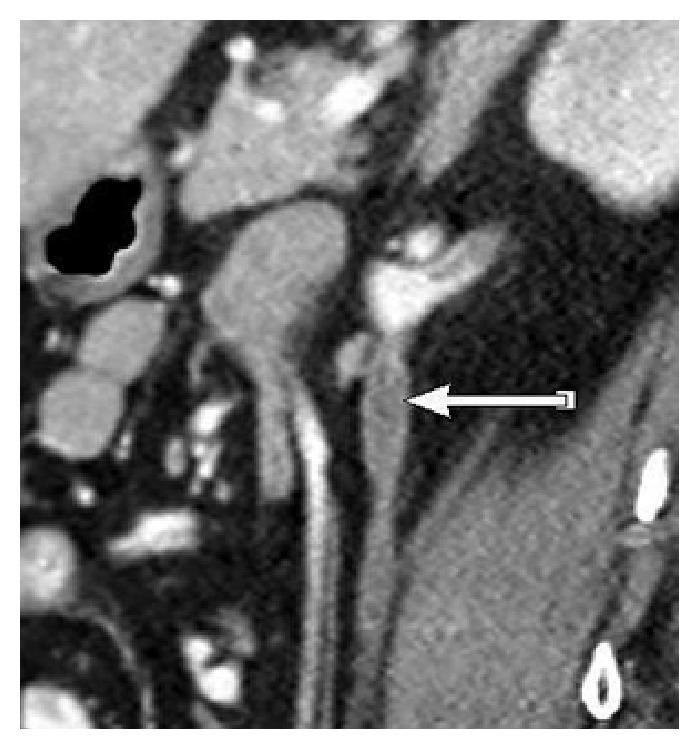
Coronal view of contrast enhanced CT in the portal venous phase, depicting enlarged right ovarian vein with central hypodense lesion, suggestive of ovarian vein thrombosis.
